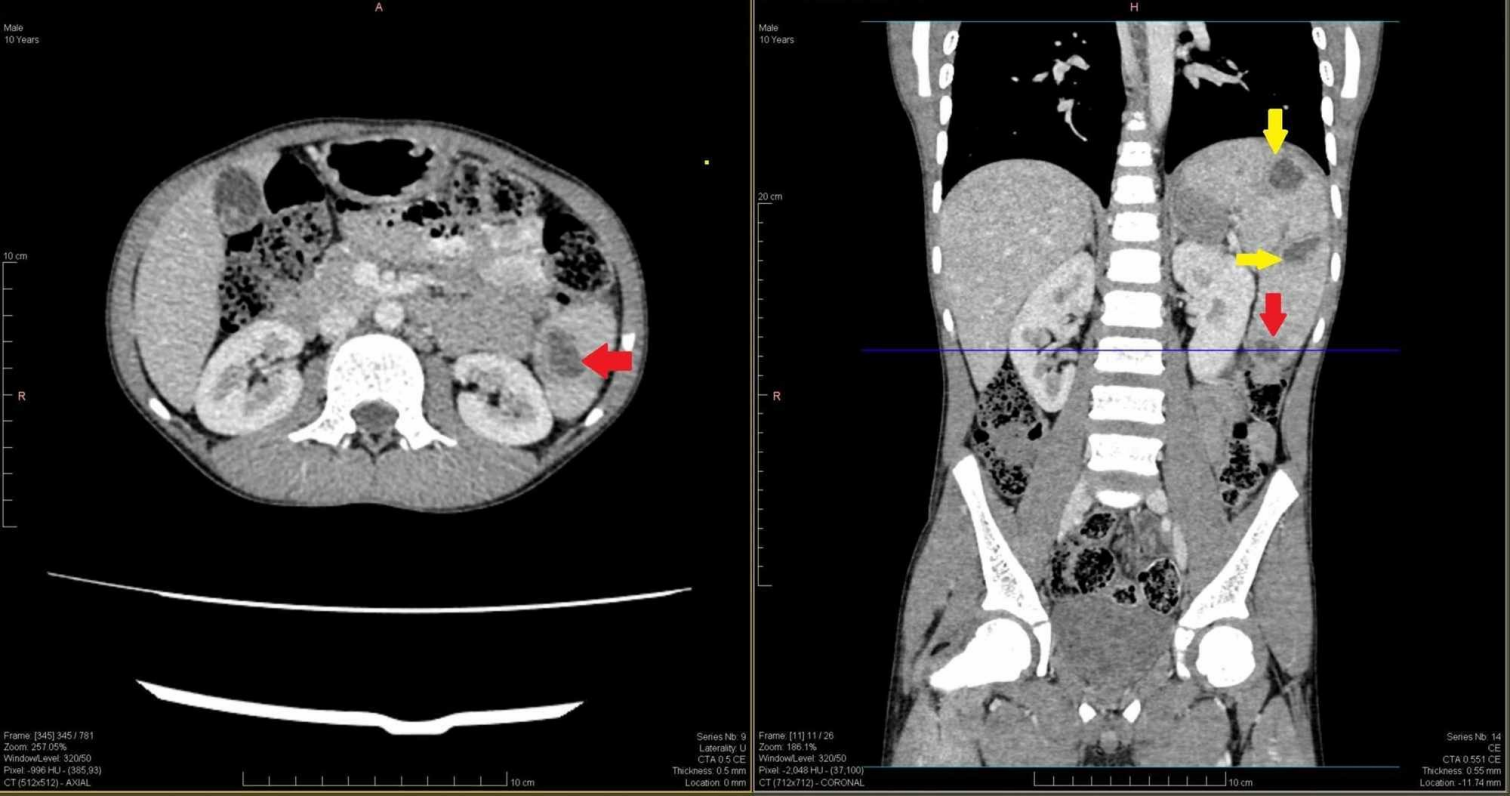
Axial and coronal views of abdominal CT scan showing multiple hypo-dense areas of hydatid cysts (red and yellow arrows), most inferior cyst (red arrow). CT: Computed tomography
